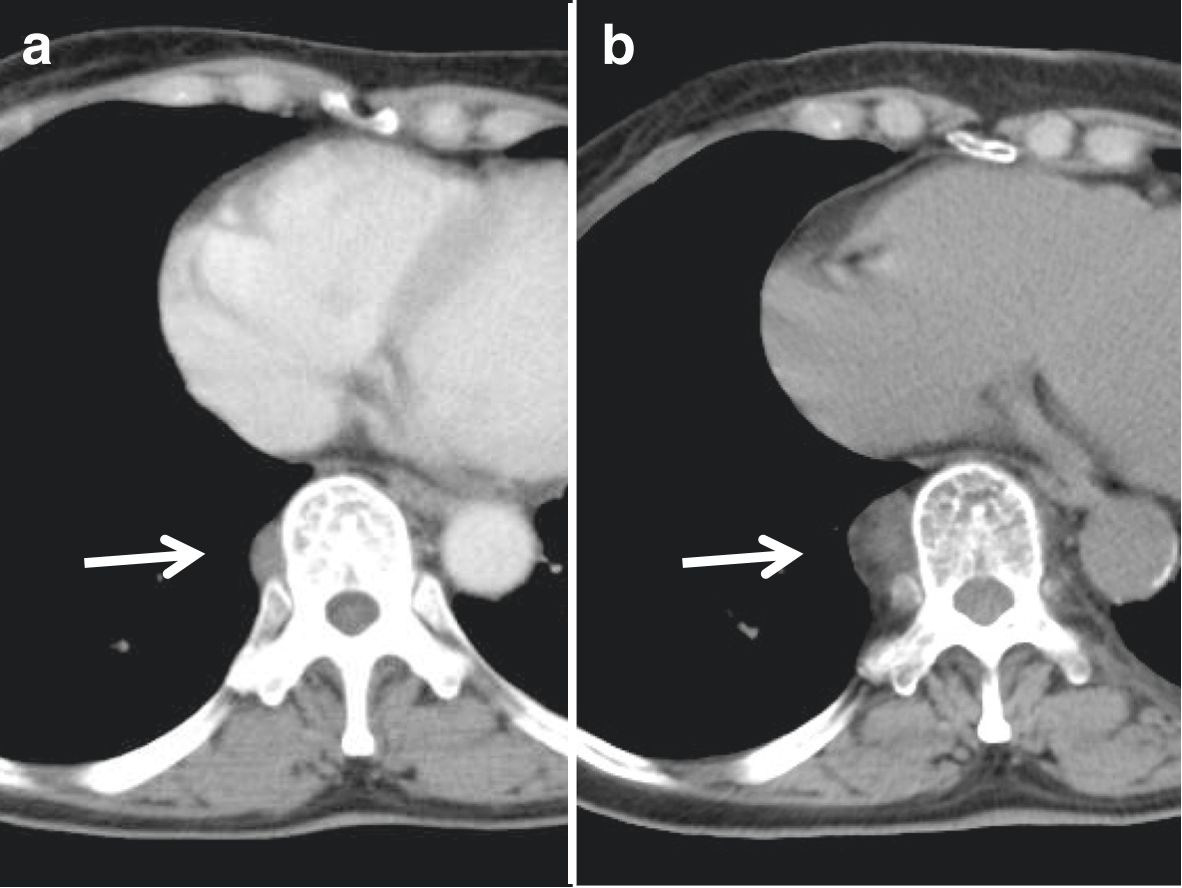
CT scan showing a 1.6 × 1.0-cm nodule 9 years previously (a), with gradual enlargement to a smooth, well-demarcated 2.8 × 2.1-cm paravertebral mass shadow, composed of a fat density area and a soft tissue density area (b)
